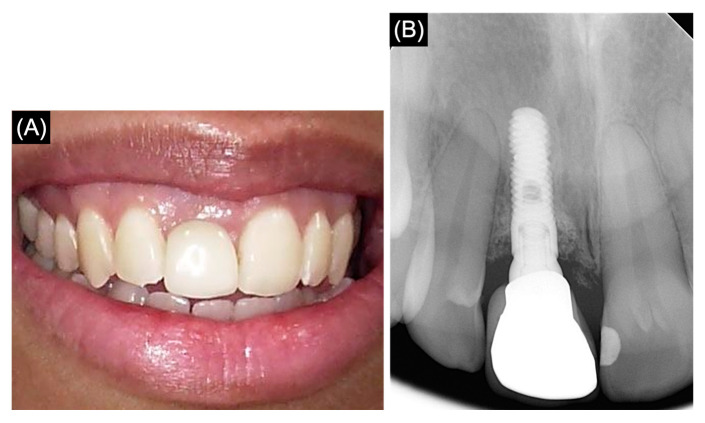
(A) Post-operative photograph of the restored single-tooth implant at site 11. (B) Periapical radiograph of the implant at site 11.
